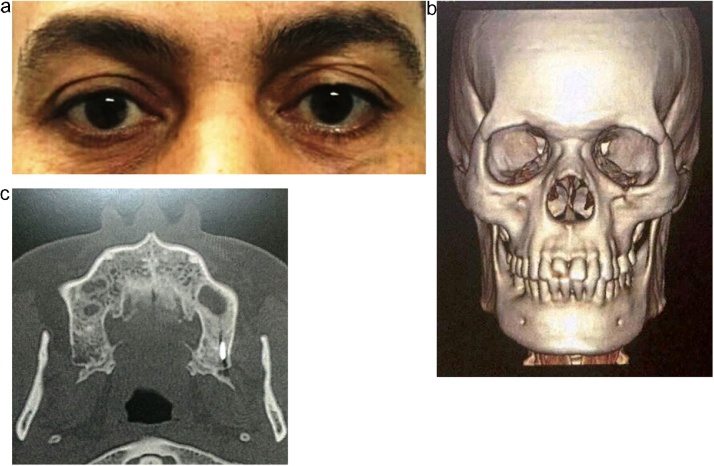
Showing clinical and radiographic results of the follow up period (a) Frontal view of the patient at 1 year postoperative with equal eye level. (b) 3D computed tomography at 2 years postoperative showing bone healing at the previous defect in the inferior orbital rim. (c) axial CT cut of the retained foreign body at 2 years of postoperative follow up.
